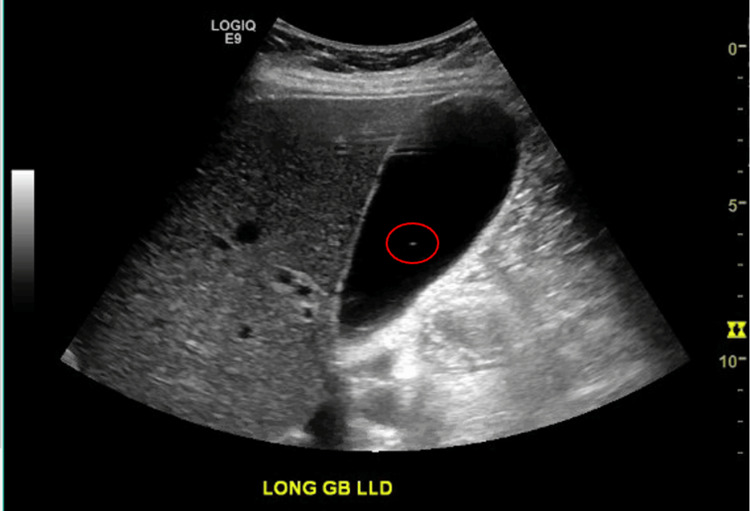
Ultrasound with a red circle showing non-obstructing gallstone
